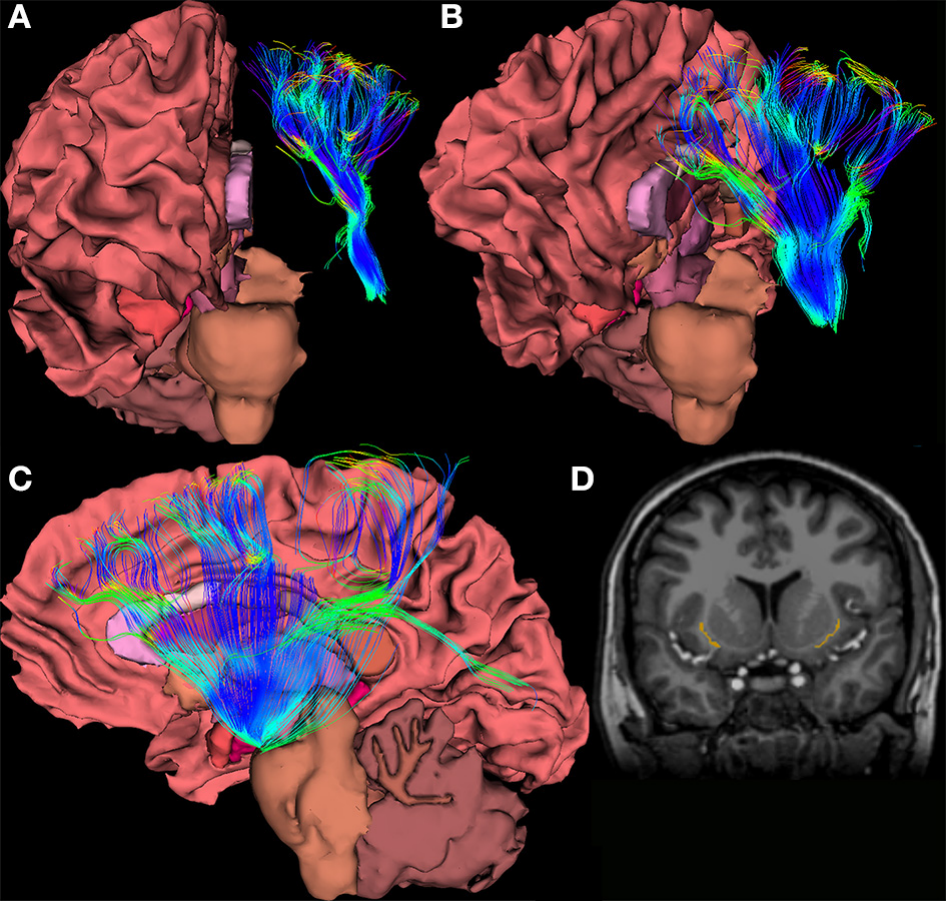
(A–C) Views of a 64-direction diffusion tensor (DTI) fiber tract reconstruction from a 3.0 Telsa Siemens MRI Trio scanner in an example subject showing white matter pathways emanating from a the region of the human claustrum. (D) The image shows a binary label mask drawn of the left and right claustra on a T1-weighted image of the same subject.
